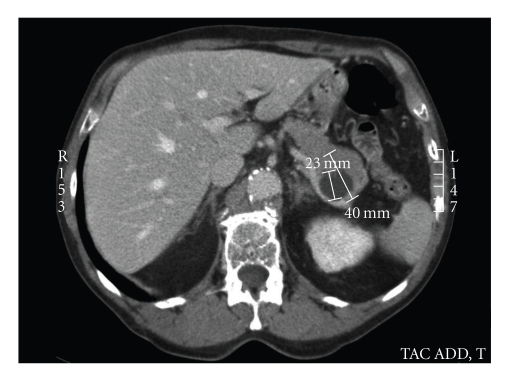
Assial CT scan showing the tumor (arrow).
